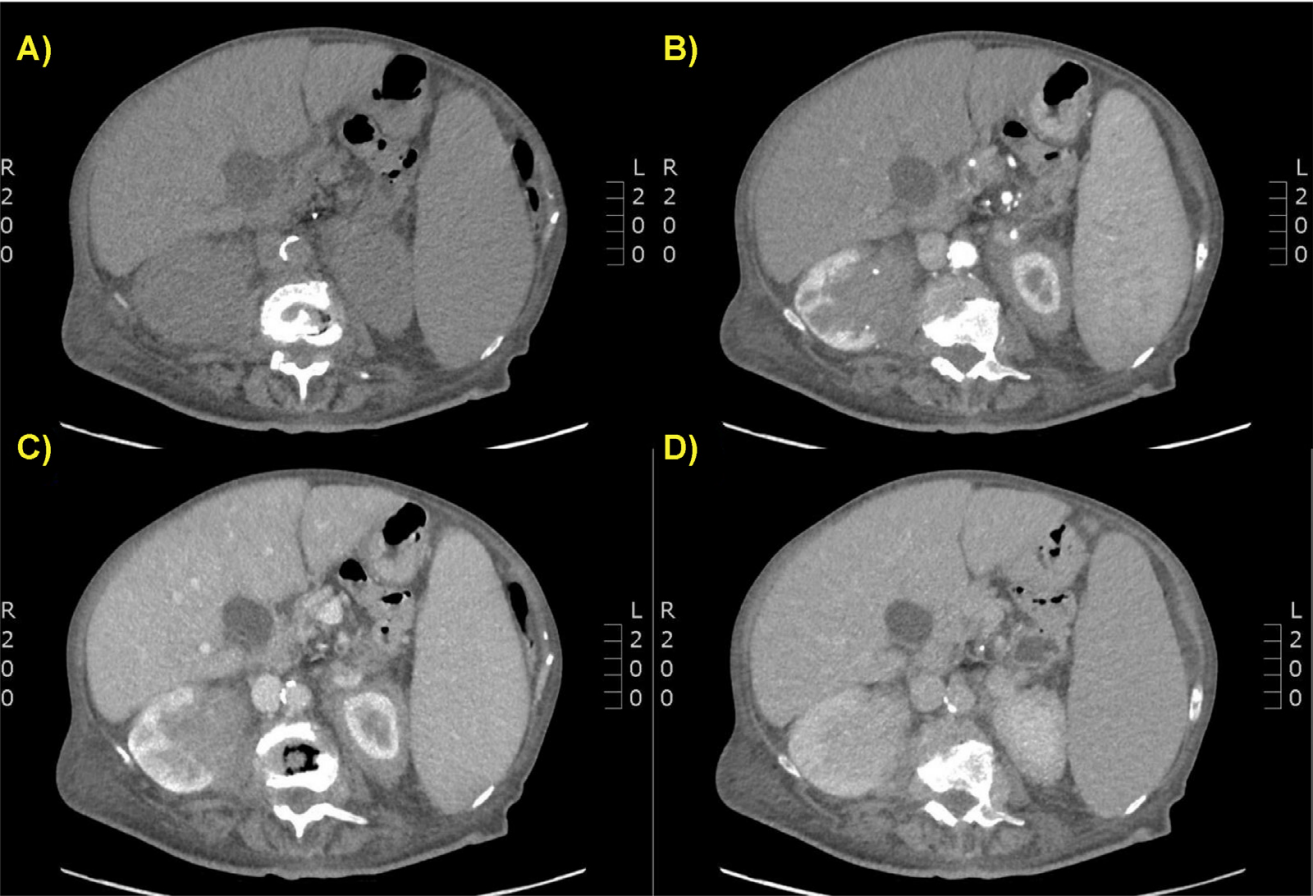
(A-D) Contrast-enhanced CT scan on axial planes. Non-contrast (A), arterial (B), venous (C), and delayed (D) phases showed altered morphology of the left kidney, partially occupied by an inhomogeneous hilar, and parenchymal renal solid area, associated with peri-renal and para-renal fluid effusion.
